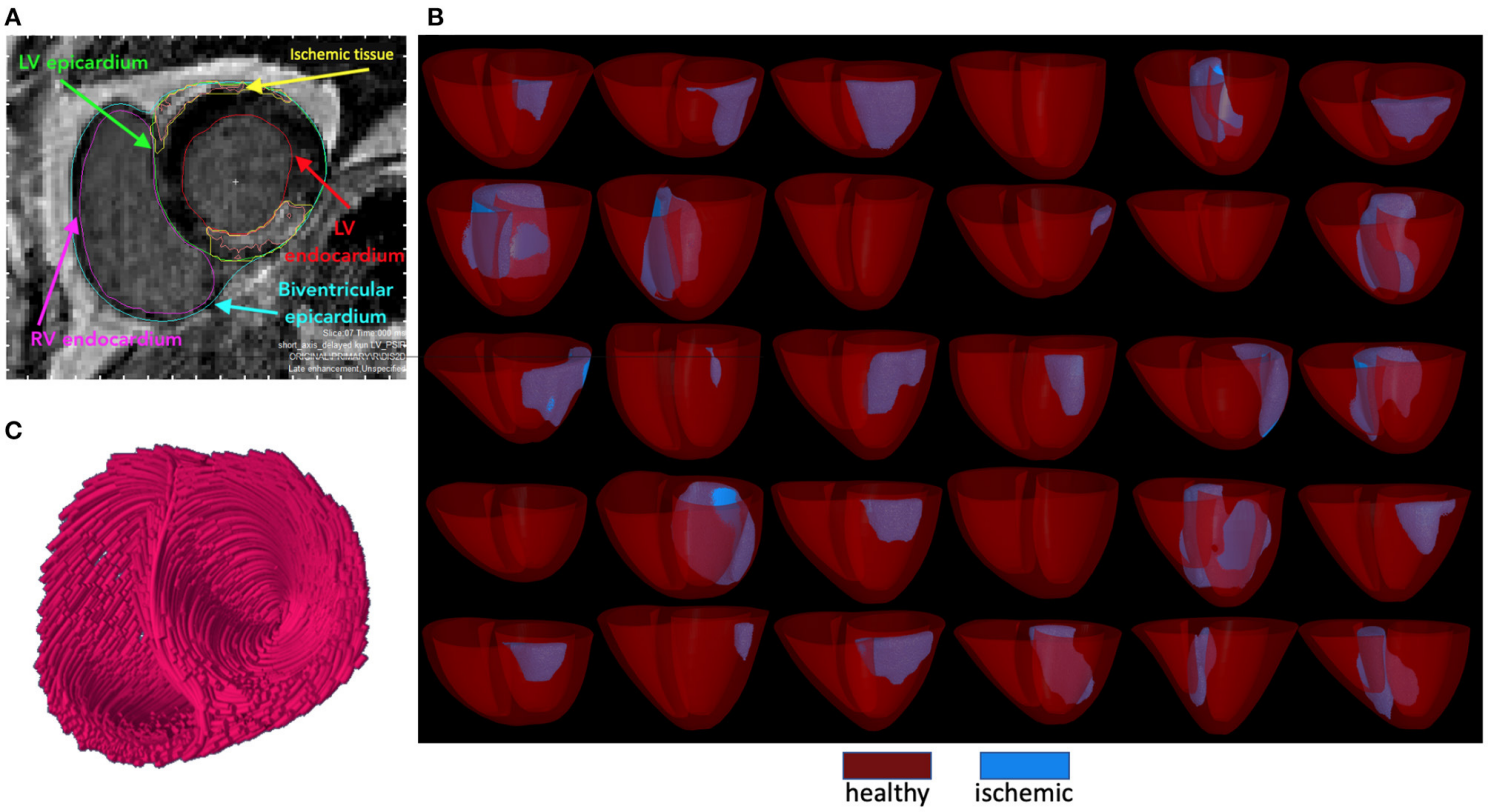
(A) Patient MRI and segmentation of endocardial, epicardial, and ischemic surfaces. (B) Rule-based fiber orientation. (C) The generated 30 baseline geometries with ventricles rendered semi-transparently.
